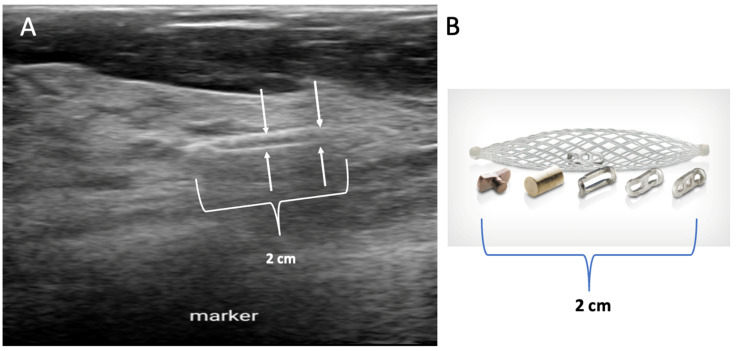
Ultrasound image (A) showing an example of a 2 cm long, basket-like, tubular, ultrasound-visible biopsy site marker (bracketed by paired arrows) that documents the location of ductal carcinoma in situ, which itself was not ultrasound visible. Image (B) shows an enlarged view of an actual basket-like marker and the shapes of various radio-opaque markers they may contain.
